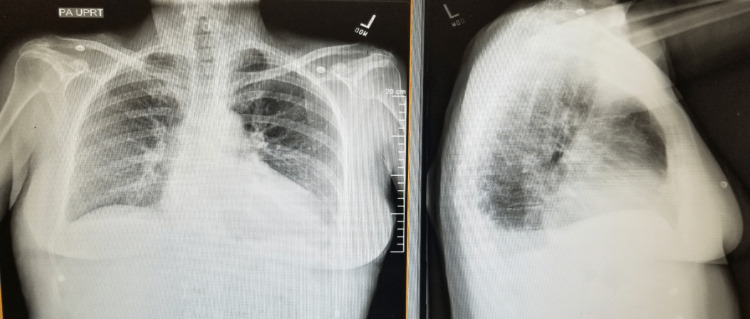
A chest X-ray at presentation demonstrating pulmonary vascular congestion.
